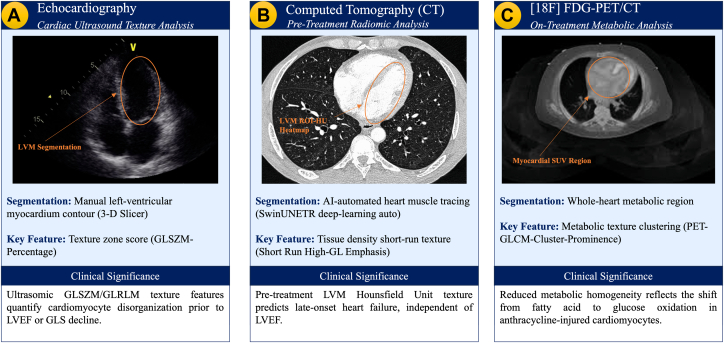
Clinical Significance of Radiomic Analysis in Cardiac Imaging (A) Ultrasomic analysis achieved from myocardial segmentation, texture feature extraction, and model output. This can aid detection of cardiomyocyte disorganization before cardiac decline. (B) CT scans often undergo deep-learning auto segmentation to extract radiomic features, which, when combined with HU texture, can predict late-onset heart failure. (C) PET/CT radiomics are extracted from myocardial SUV data and whole-heart metabolics, with cardiac homogeneity showing clinical significance (PET/CT, 95% accuracy).^73^^,^^85^ CT = computed tomography; FDG = Fluorodeoxyglucose/18F-FDG; LVEF = left ventricular ejection fraction; GL = gray Level; GLCM = gray level co-occurrence matrix; GLRLM = gray level run length matrix; GLS = global longitudinal strain; GLSZM = gray level size zone matrix; HU = Hounsfield units; LVM = left ventricular myocardium; PET = positron emission tomography; ROI = region of interest; SUV = standardized uptake value.
